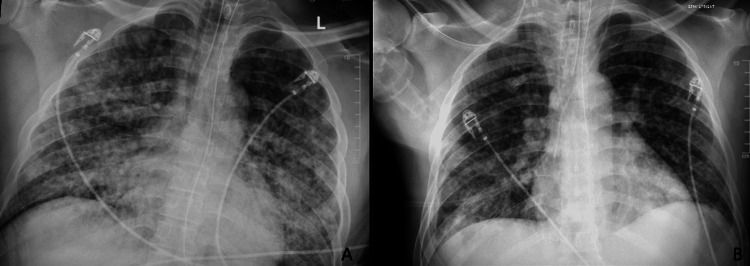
Improvement in bilateral consolidation after treatment of disseminated varicella-zoster virus (VZV) Plain films on admission (A) and 12 days after admission (B), showing greatly improved bilateral consolidation.
